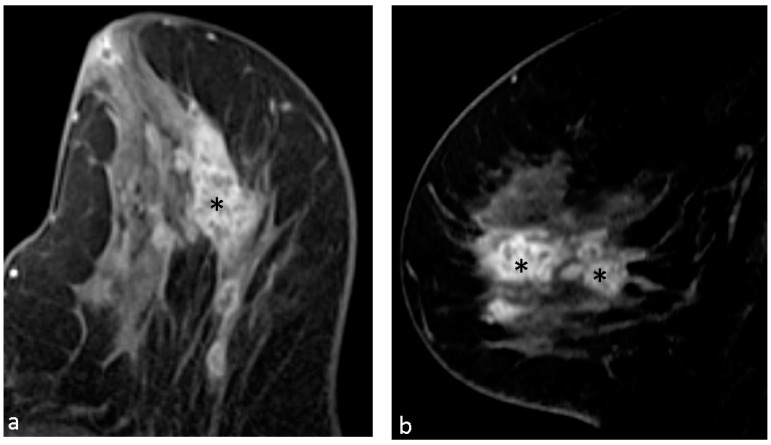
A 77-year-old woman with left breast mastalgia for one week. Axial (a) and sagittal (b) T1-weighted contrast-enhanced MR imaging shows clustered ring NME lesion (*) with segmental distribution. Pathological results of left breast revealed infiltrating duct carcinoma and ductal carcinoma in situ.
